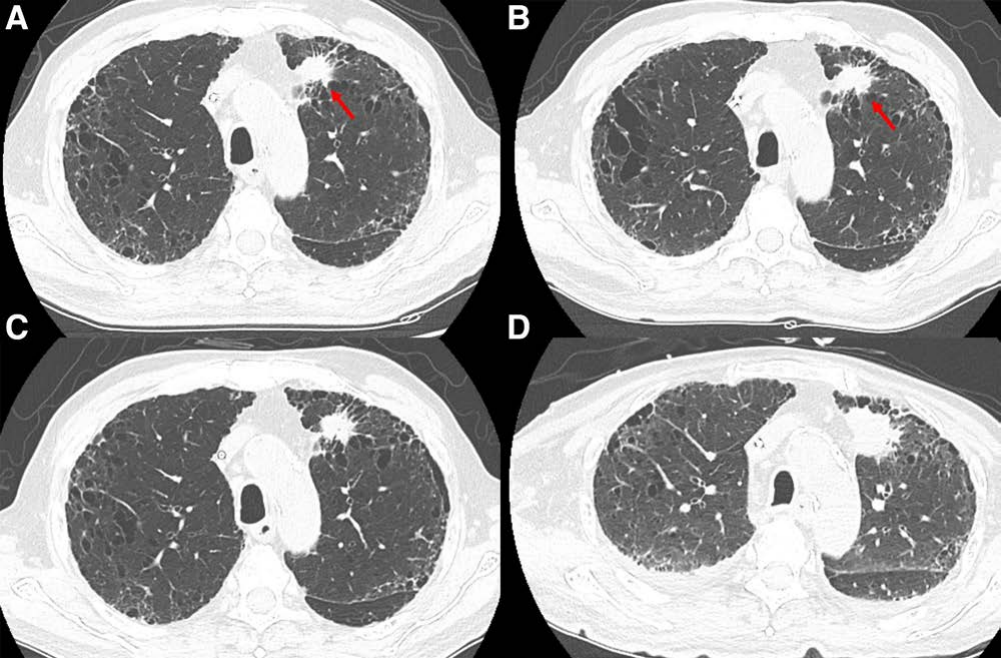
Changes in lung tumor at initial diagnosis and postchemotherapy. (A) Baseline CT scan of the chest before initiating treatment with nivolumab; (B) CT scan after 5 cycles of nivolumab (SD according to RECIST version 1.1); (C) CT scan after 9 cycles of nivolumab (SD); and (D) CT scan 4 mo after nivolumab discontinuation (progressive disease). CT = computed tomography, RECIST = Response Evaluation Criteria in Solid Tumors, SD = stable disease.
